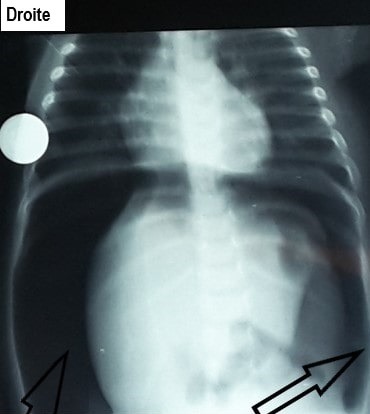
Radiographie de l’abdomen sans préparation; pneumopéritoine massif, absence de niveau hydroaérique au sein de l’estomac et une faible pneumatisation intestinale
